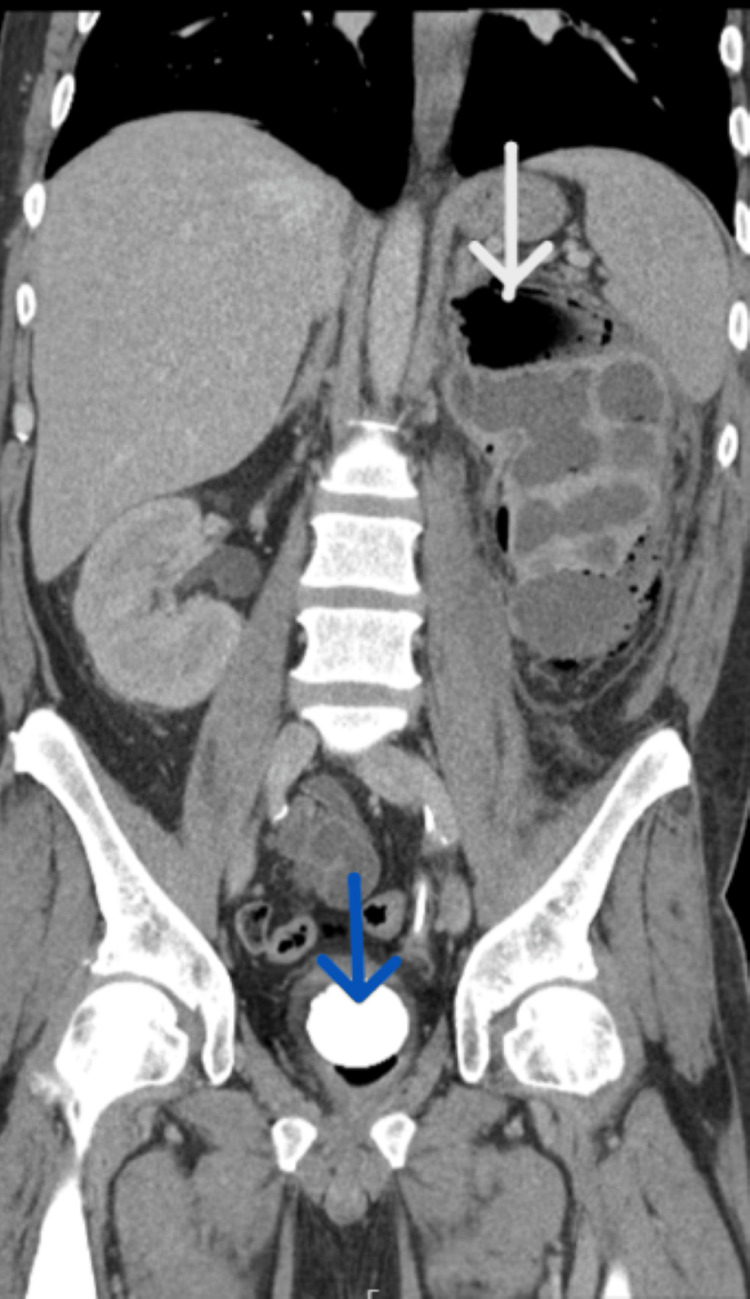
Coronal view of the CT scan shows an enlarged left kidney with areas of fluid collection (white arrow) and a 5.6 cm bladder stone (blue arrow).
